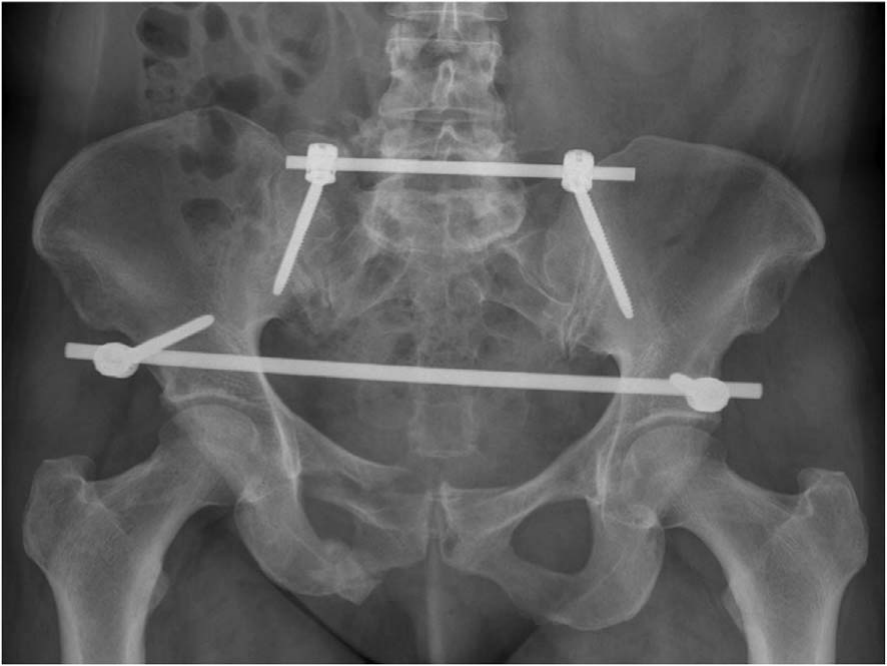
Postoperative plain radiograph demonstrated complete pelvic ring fracture at the right side with a sacral fracture treated with a TIFI and an anterior internal fixator.
